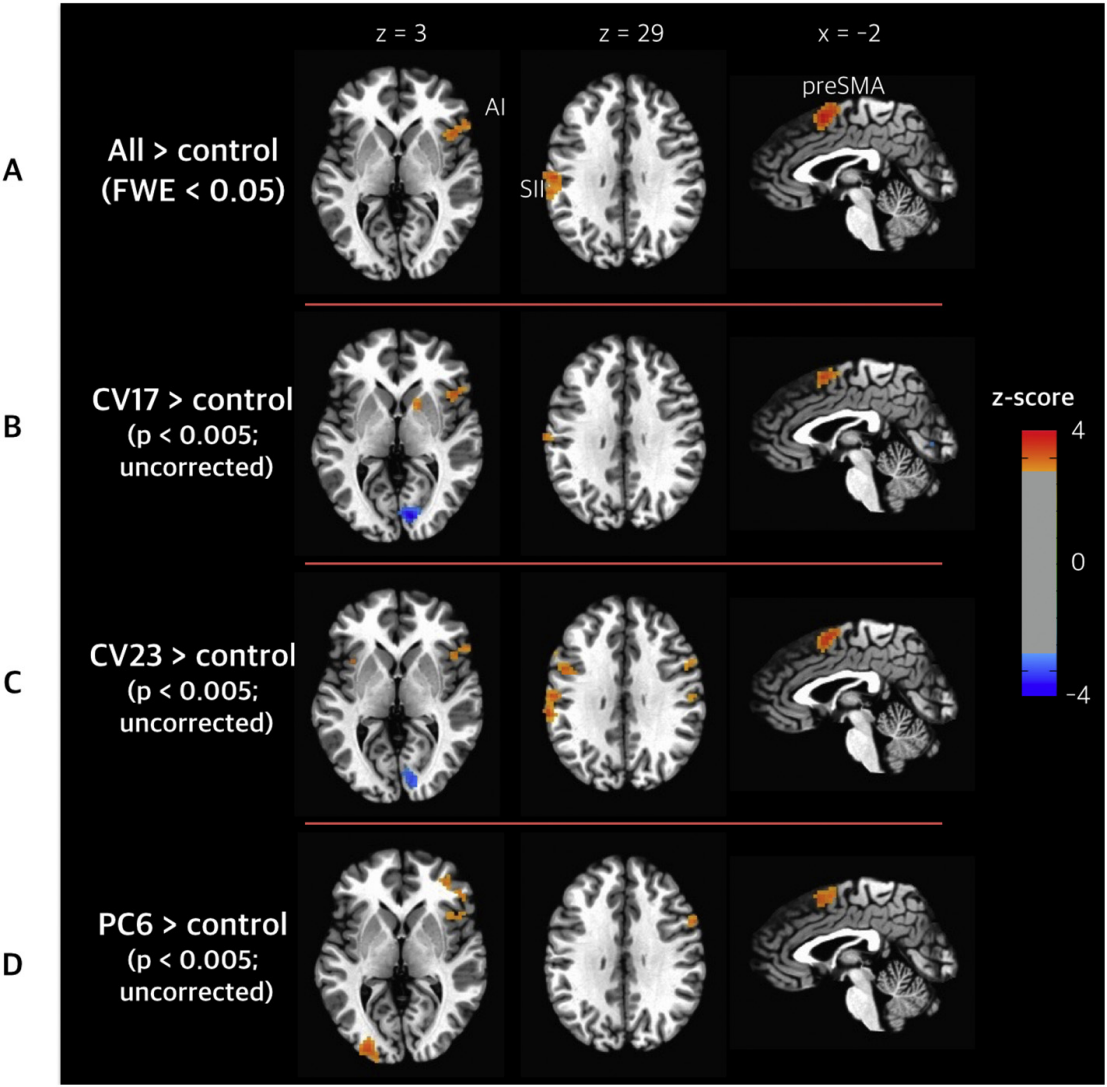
Psychophysiological responses to the cognitive component of cutaneous electrical stimulation. All three pseudo-stimulations commonly exhibited brain activation in the right anterior insula, right inferior frontal gyrus (rIFG), pre-supplementary motor area (pre-SMA), and secondary somatosensory cortex (SII) (*p* < .05, FWE-corrected). The common functional magnetic resonance imaging activation map (A) for pseudo-stimulation and the activation pattern of each condition (B, C, and D) in the same brain section. Statistical t-maps of all acupoint conditions over the control condition are overlaid on an average structural image, and the significance threshold was set to *p* < .005 (uncorrected) for visualization purposes only.
